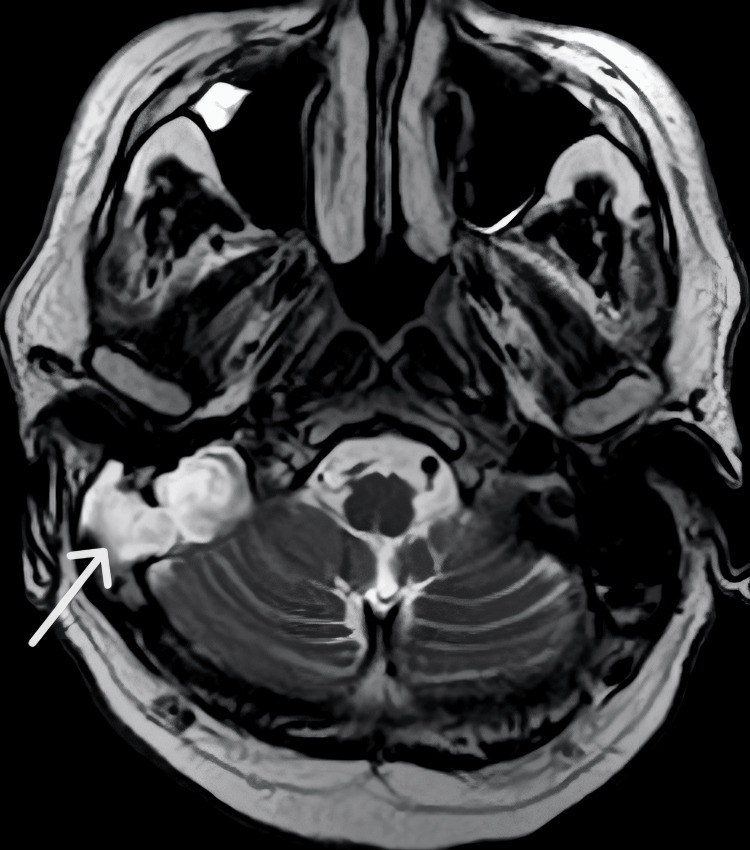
Axial T2-weighted MRI demonstrating a hyperintense lesion in the right temporal bone (white arrow), corresponding to the mastoid region, without evidence of intracranial extension
